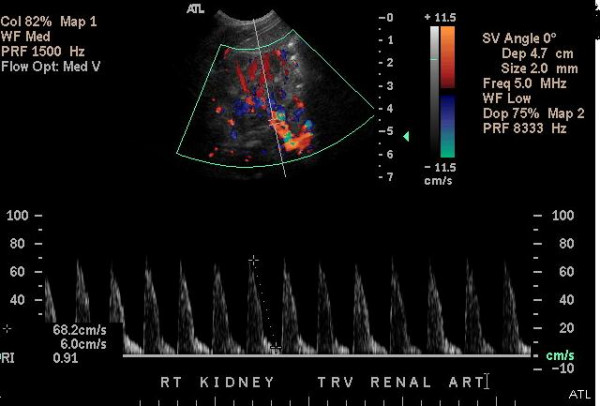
Renal Doppler flow study demonstrating an increased resistive index of the right renal artery with suboptimal wave forms of the right renal vein.
